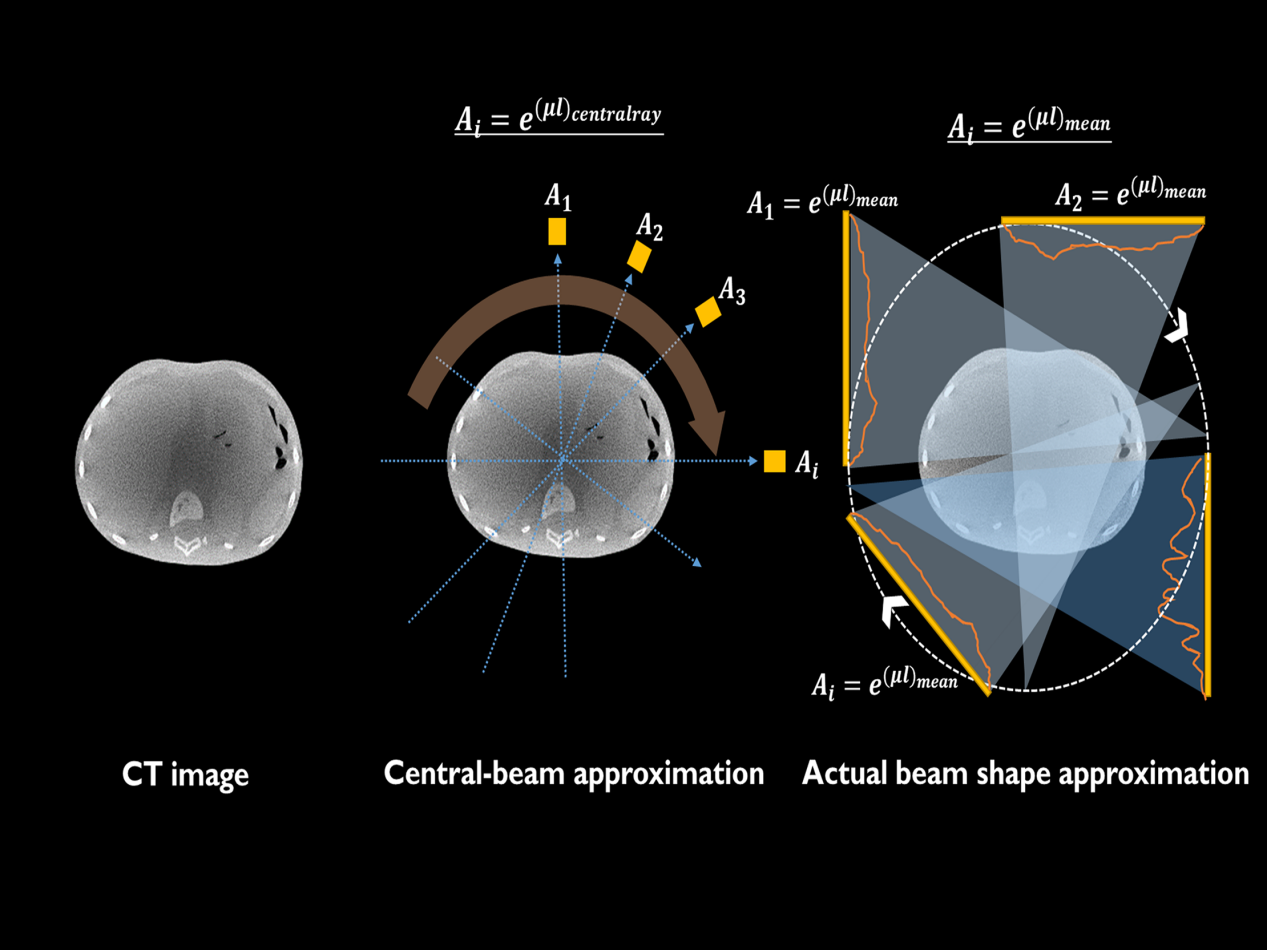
Framework for calculating Ai values. After selecting the CT image as in (a), we calculated the Ai value by two methods (b) Central-beam approximation and (c) actual beam shape approximation.
